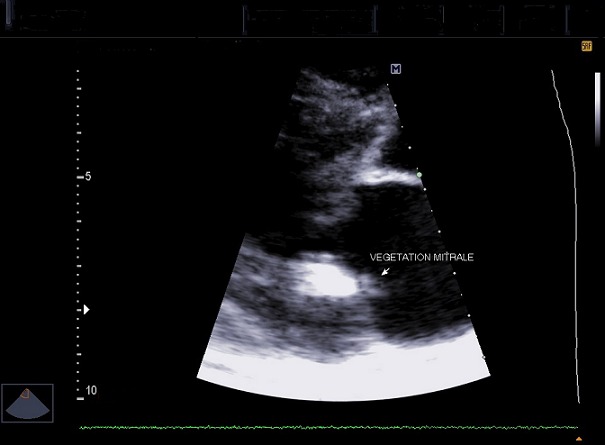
Échocardiographie doppler cardiaque réalisée à J14 d’hospitalisation montrant de végétation au niveau petite valve mitrale
